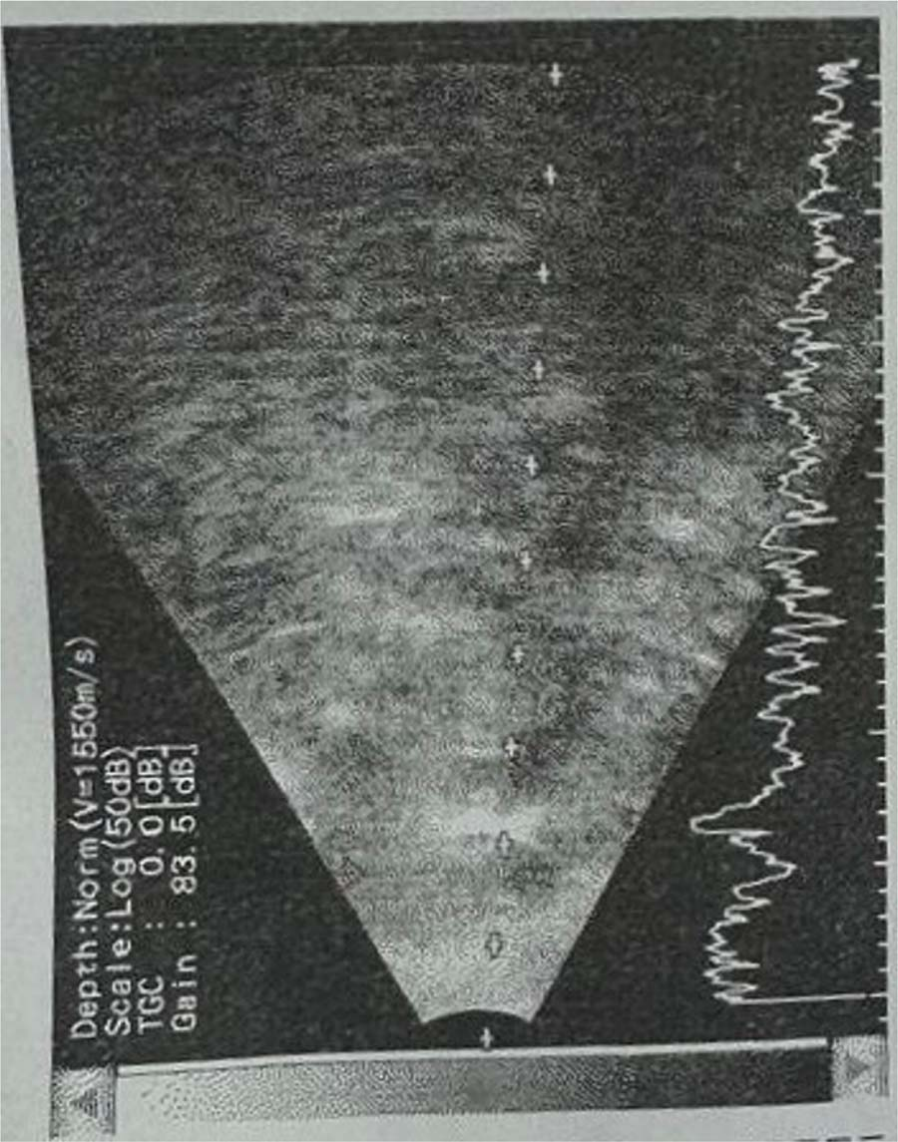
B-scan ultrasound showing sever vitreous echoes and vitreous abscess in patient 5.
